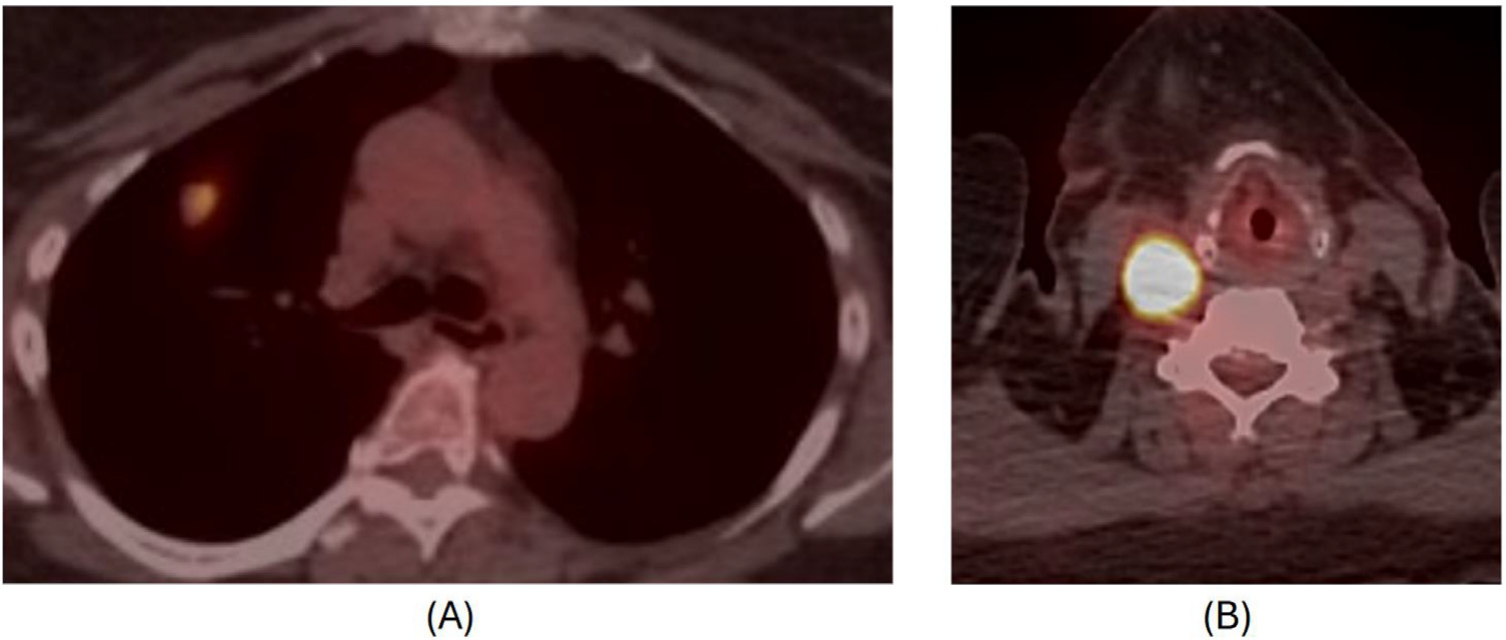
(A, B): Initial PET CT axial image demonstrating hypermetabolic solid lung nodule measuring up to 1.7 cm (A). Hypermetabolic mass was incidentally identified near the right carotid bifurcation (B).
